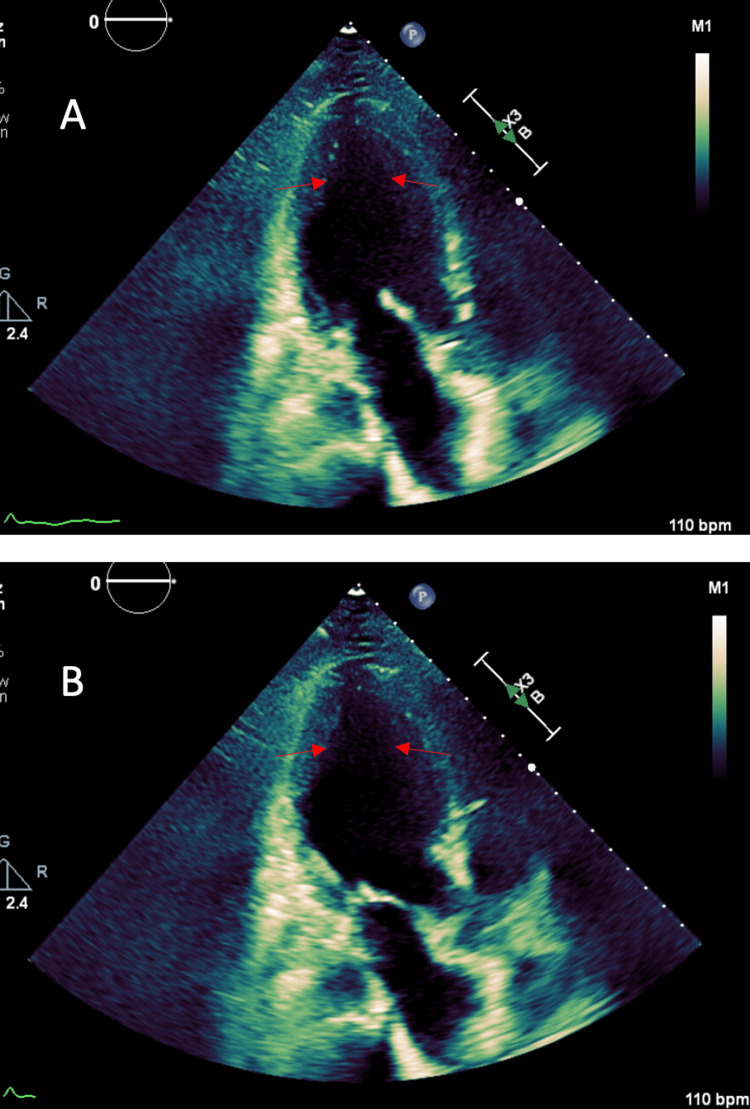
Echocardiography in (A) end-diastolic phase and (B) end-systolic phase on admission. Arrows point to ventricular wall abnormalities.
